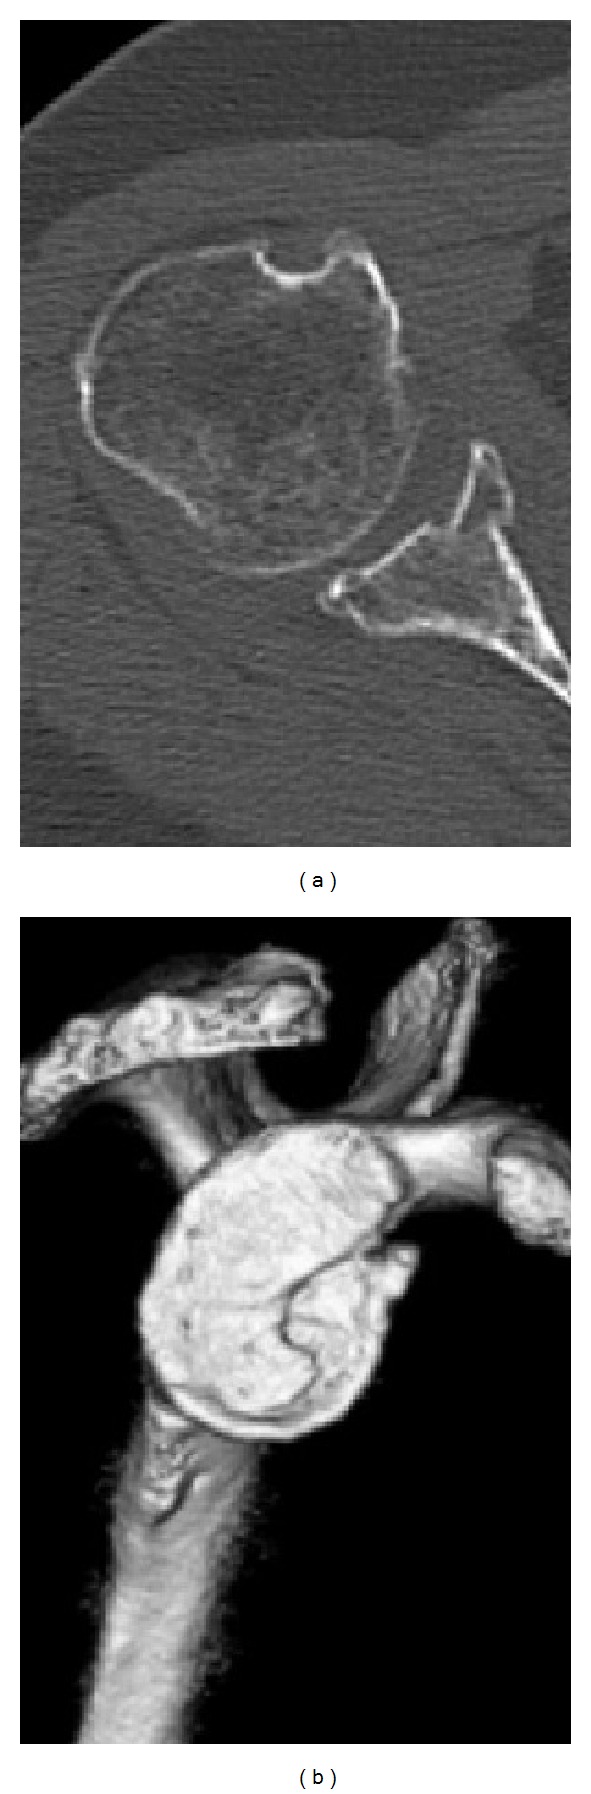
CT imaging upon initial presentation at our hospital. (a) Axial CT imaging showing a large glenoid rim fracture and an articular step-off of 2.5 mm. (b) The width of the bony fragment was 30% of the glenoid length on the three-dimensional CT.
